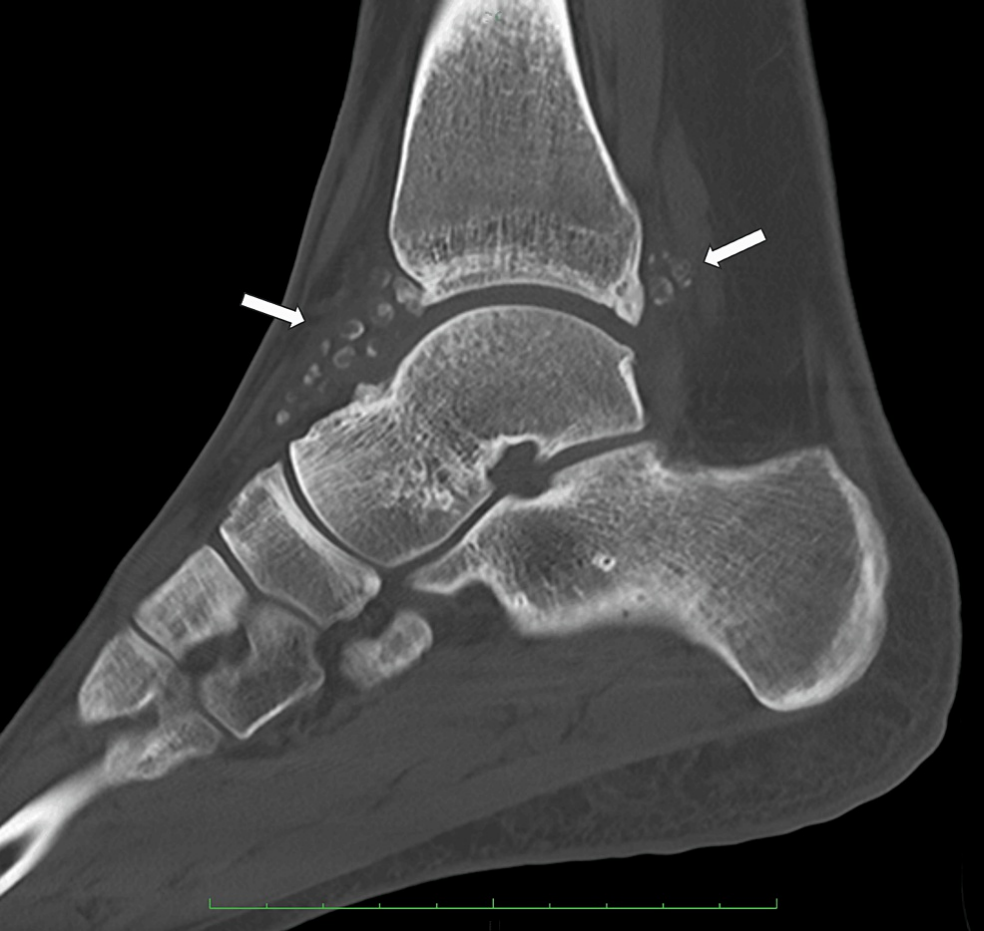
Preoperative sagittal CT of the right ankle.
